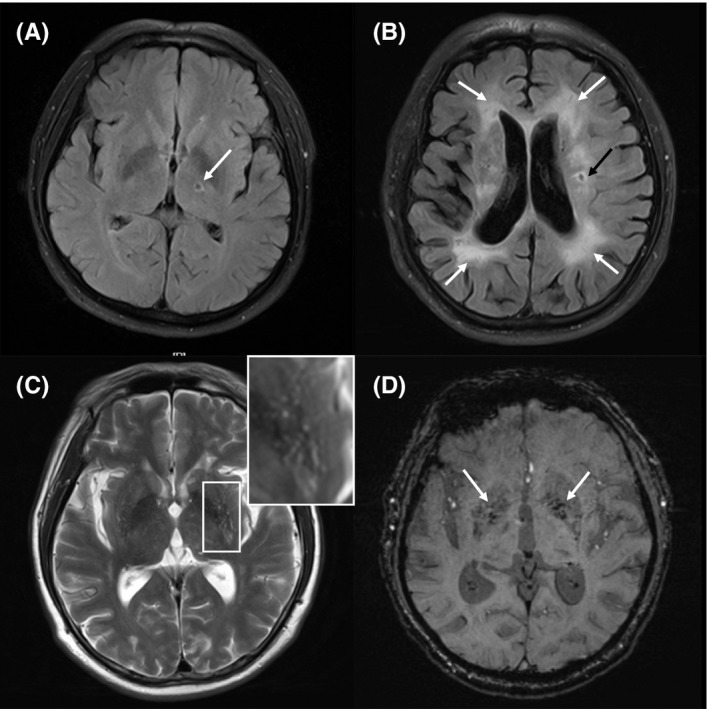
Representative MRI features of CSVD. (A) Lacune (arrow) on fluid‐attenuated inversion recovery (FLAIR) imaging. (B) White matter hyperintensities (white arrows) accompanied by lacune (black arrow) on FLAIR imaging. (C) Perivascular spaces on T2‐weighted imaging. (D) Cerebral microbleeds (arrows) on susceptibility‐weighted imaging (SWI)
